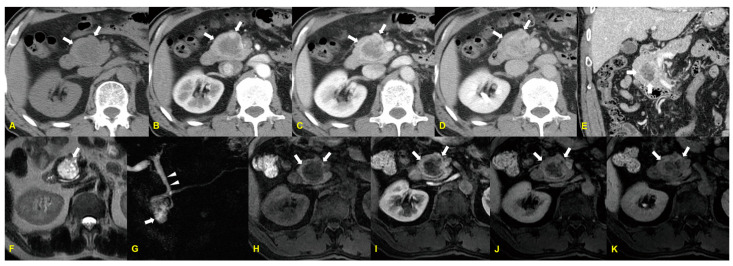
A 65-year-old man with colloid carcinoma. The precontrast axial CT image (A) shows a low attenuated mass in the pancreatic head (arrows). Contrast-enhanced arterial (B), portal (C), delayed (D) phase, and coronal reformation (E) CT images demonstrate peripheral enhancement on arterial phase images and internal mesh-like enhancement on portal and delayed phase images (arrows in (B–E)). T2-weighted MR (F) and 3D MR cholangiopancreatography (G) images demonstrate typical salt-and-pepper appearance (arrow in (F,G)) and the absence of communication of the mass with the pancreatic duct. The mass caused mild dilatation of the common bile duct (arrowheads in (G)). The unenhanced T1-weighted MR image (H) and gadolinium-enhanced arterial (I), portal (J), and delayed (K) phase T1-weighted MR images show a progressively peripheral and internal sponge-like enhancement pattern (arrows).
